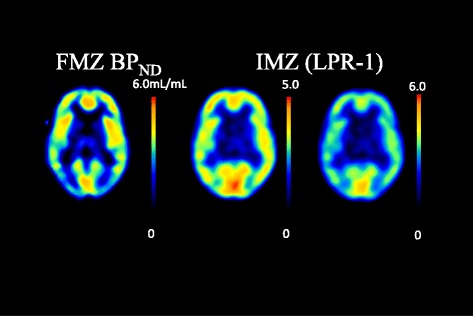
Parametric images of FMZ PET and IMZ SPECT. *Left* is FMZ BP_ND_ (0–6.0 mL/mL). *Center* image is IMZ (LPR-1) ranging from 0 to 5.0. *Right* image is IMZ (LPR-1) ranging from 0 to 6.0
